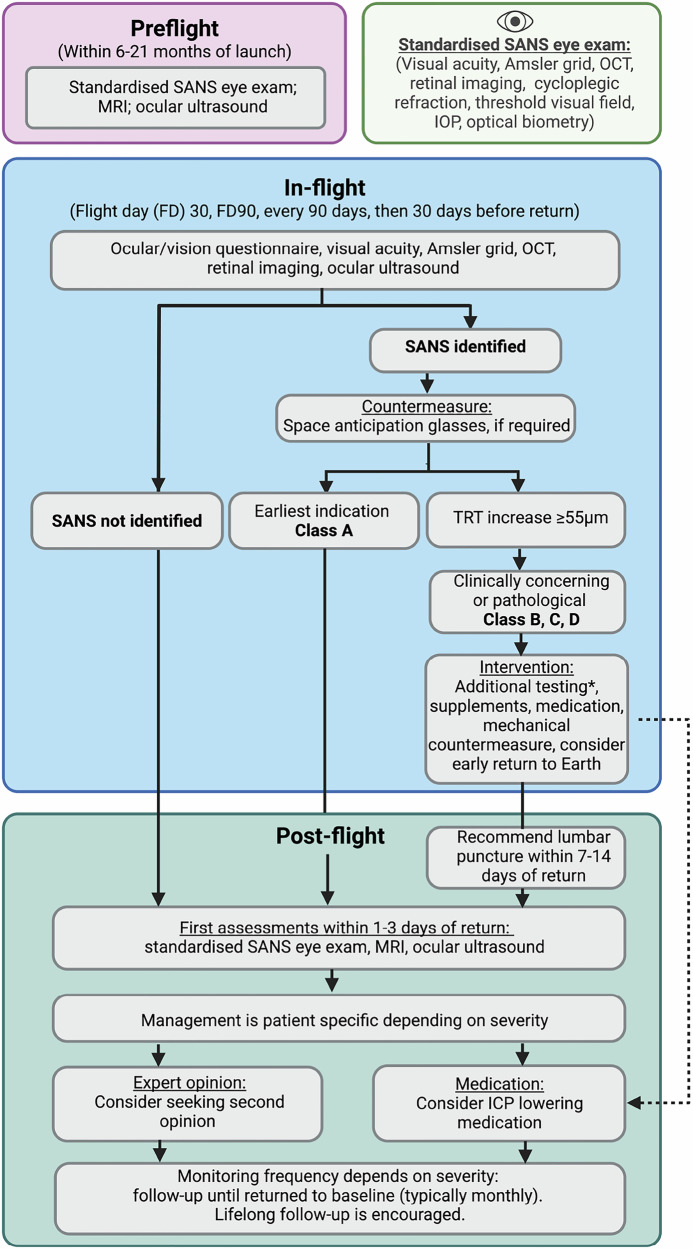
Consensus management overview for spaceflight neuro-ocular syndrome (SANS). Adapted from the primary testing and decision tree overview for spaceflight-associated neuro-ocular syndrome (SANS) as applied to NASA astronauts assigned to long-duration missions onboard the International Space Station (ISS). MRI magnetic resonance imaging, FD flight day, OCT optical coherence tomography, TRT total retinal thickness, IOP intraocular pressure, ICP intracranial pressure. *More frequent assessments may be required, depending on severity or rate of progression.
